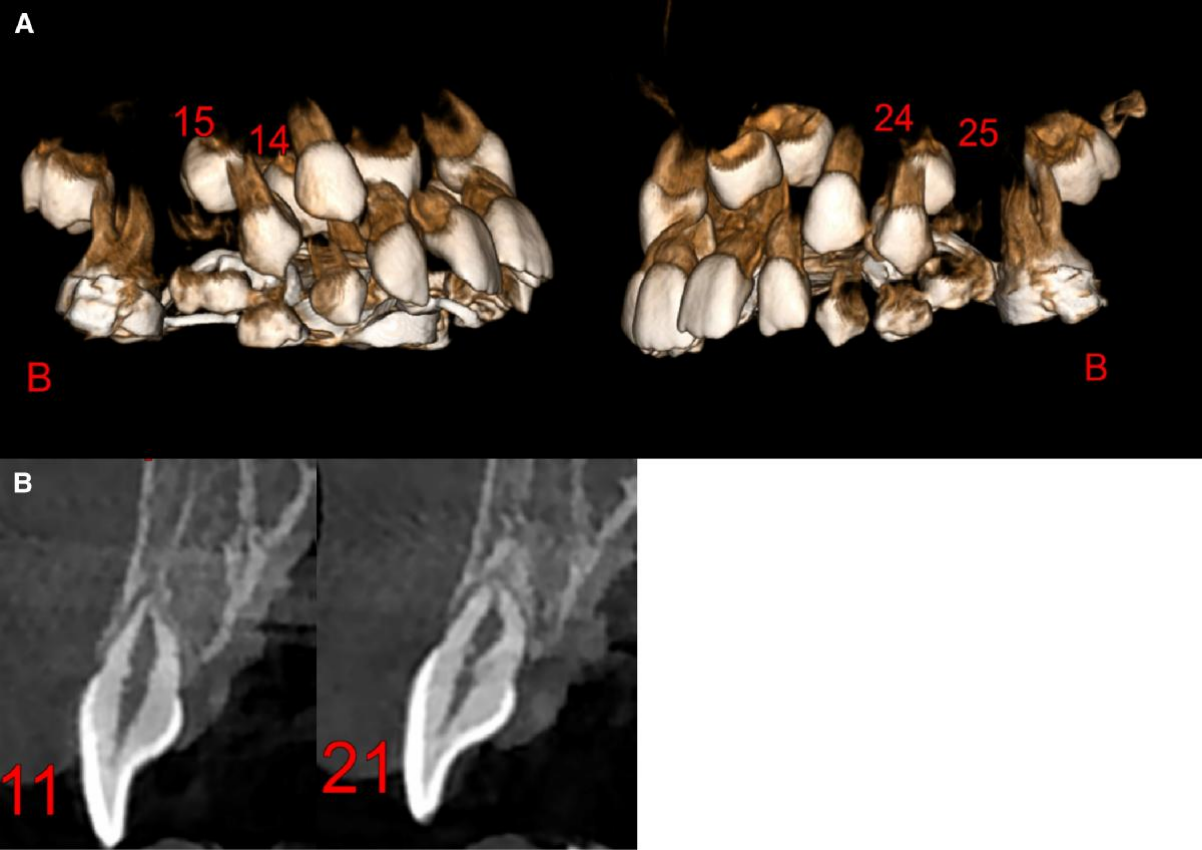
Cone beam imaging of a heterozygous *PRKAR1A* variant c.379_380delinsTT; p.Ala127Phe in Individual 1, Family 12, TS1. Volume rendering of the upper dental arch A) and buccolingual slices passing, respectively, through the upper central incisors (#11–21). Transposition tendency of germs #15 and #25; B) root abnormalities with short root portions of teeth #11–21.
